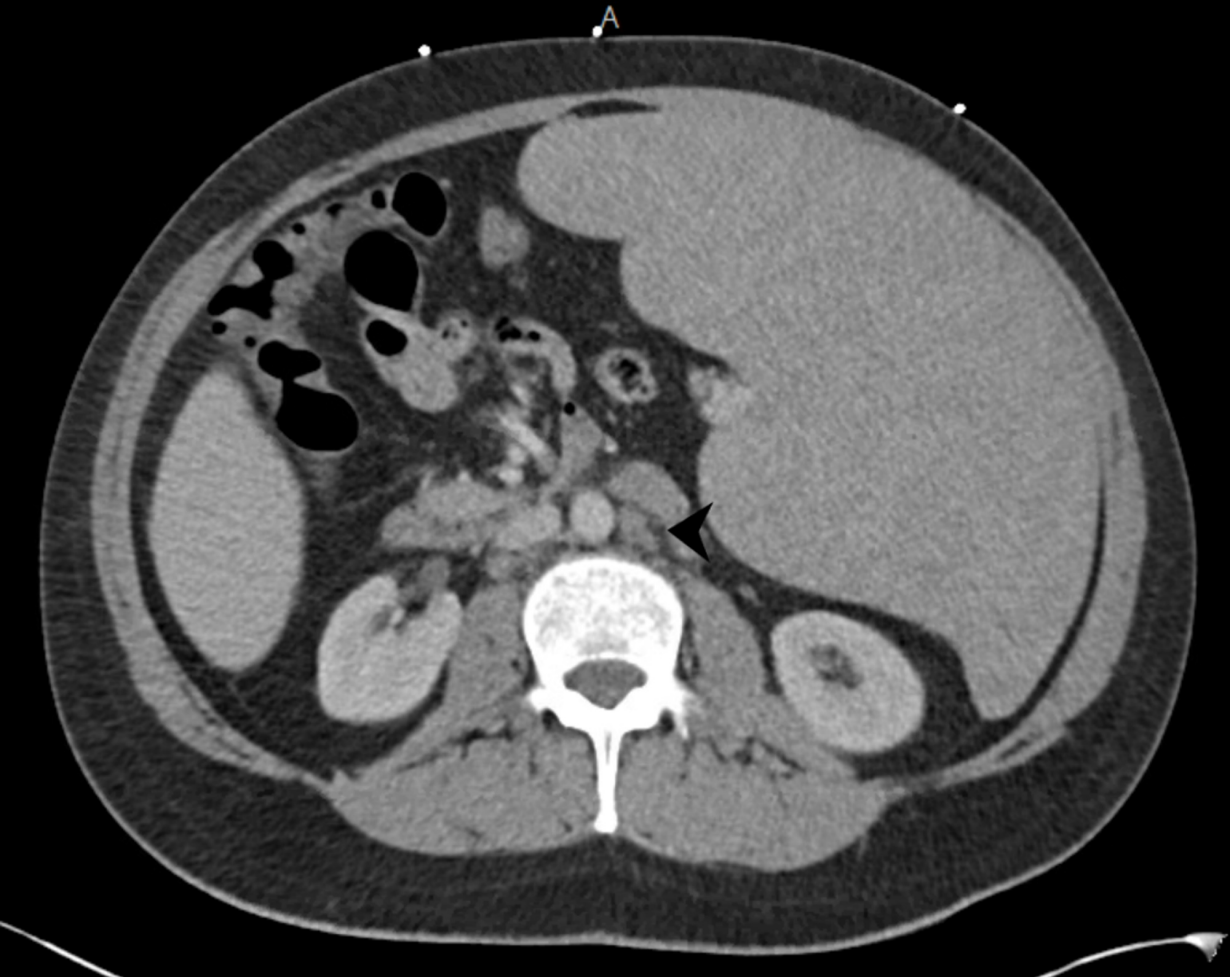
CT abdomen and pelvis with IV contrast demonstrating a 1.4 x 2.5 cm left para-aortic lymph node.
